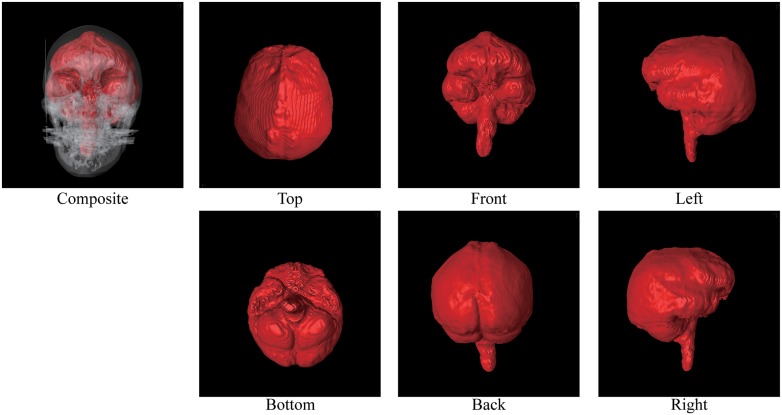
Results of endocast extraction (CT Head).
